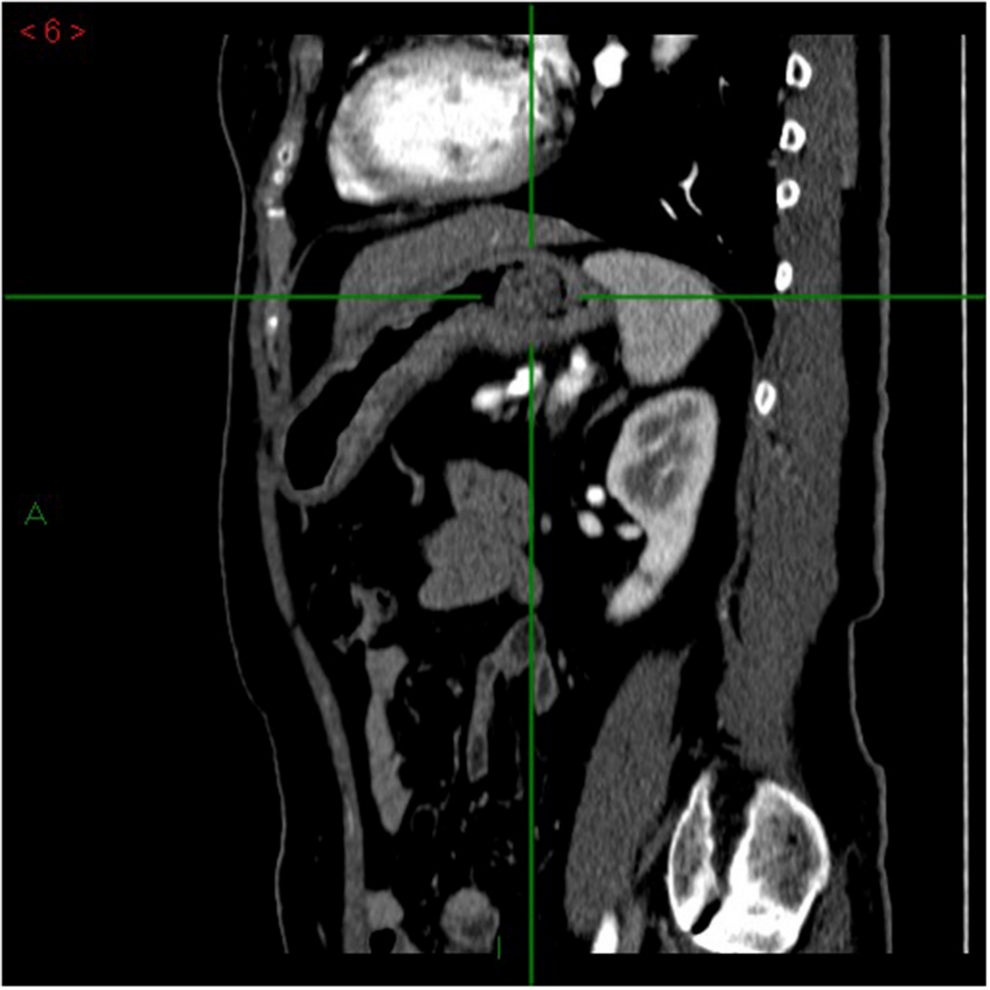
Multidetector computed tomography (MDCT) arterial-phase, sagittal plane. Focal mass in the gastric lumen, next to the cardias, characterized by enhancement comparable to that of the gastric wall. The mass is vegetating into the gastric lumen.
